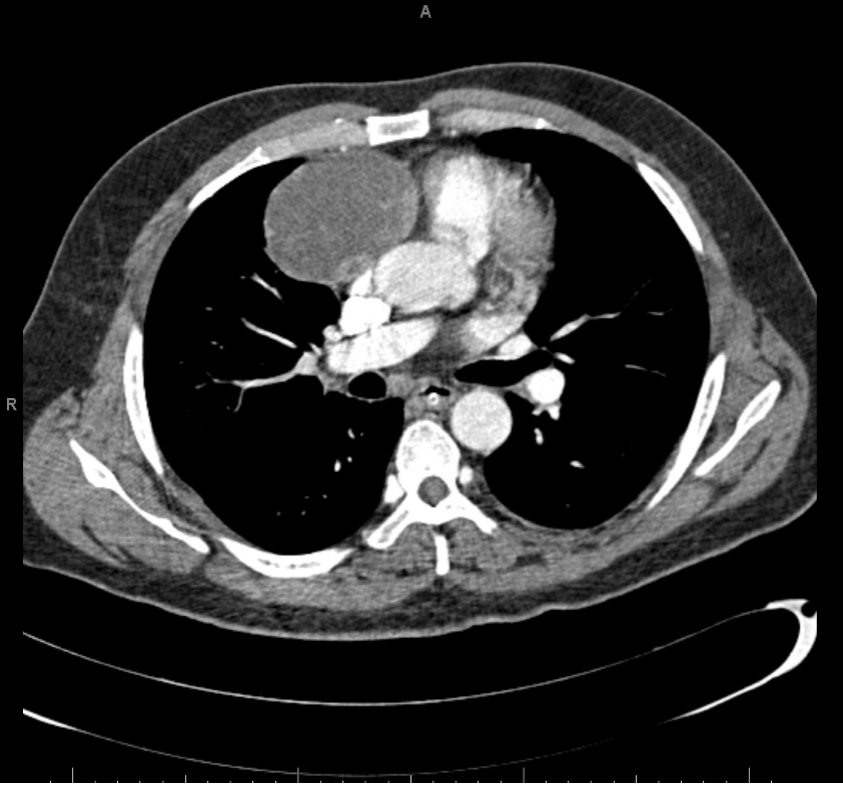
A computed tomography scan of the patient’s chest showing an anterior mediastinal mass.
